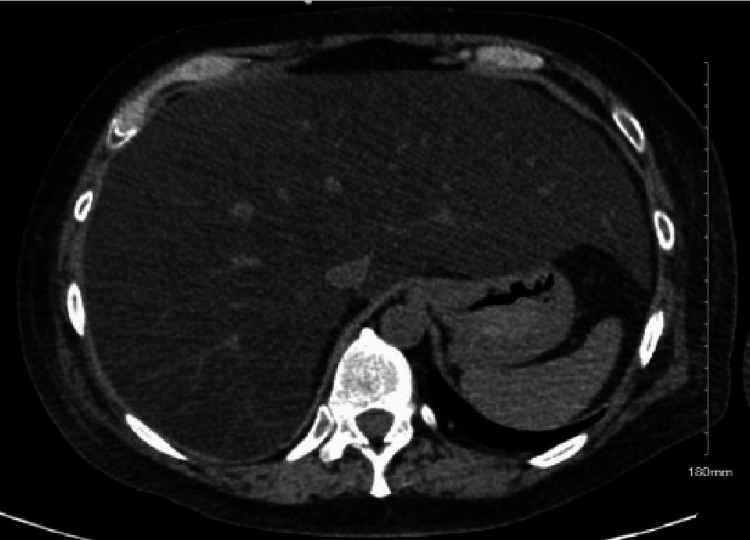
CT abdomen revealing hepatomegaly.
